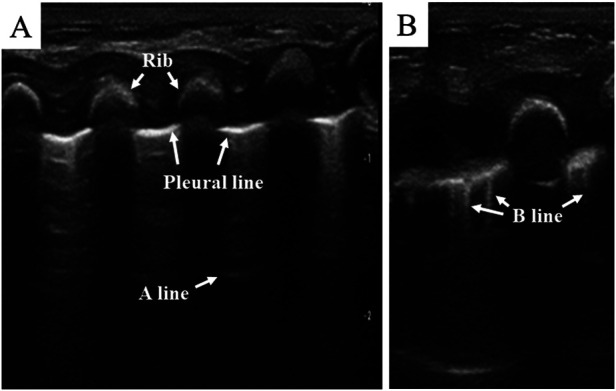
Normal lung pattern on lung ultrasonography. (A) Pleural line: the pleura is visualized as a smooth, regular echogenic line; A-lines: equidistant, horizontal, and echogenic lines distal to the pleural line. (B) B-line: vertical hyperechoic artifacts arising from the pleural line.
